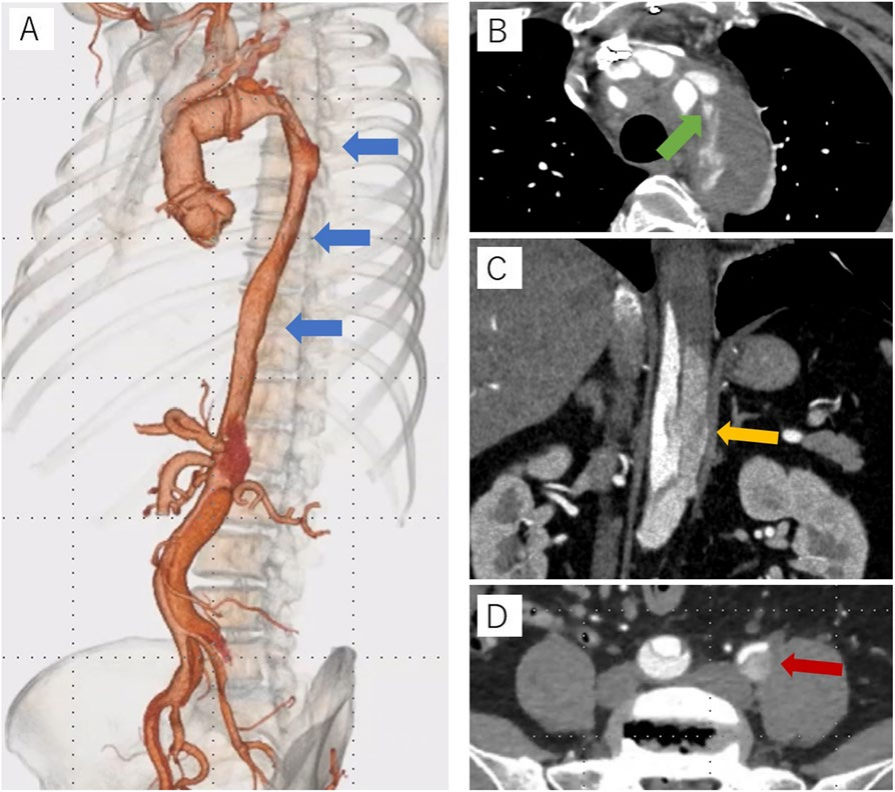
A–D CT images before STABILISE technique. Legend: A 3D image of the CT scan 9 days after the proximal aortic repair. True lumen of the descending thoracic aorta was narrowed due to compression by the false lumen (blue arrow). B Axial image at the level of the left SCA ostium. Residual dissection in the left SCA along with antegrade blood flow into the false lumen of the aortic arch was observed (green arrow). C Coronal image of the thoracoabdominal aorta. Retrograde blood flow into the false lumen of the thoracoabdominal aorta to descending aorta was observed (yellow arrow). D Axial image at the level of the EIA. The true lumen of the left EIA is narrowed due to compression by the false lumen (red arrow)
